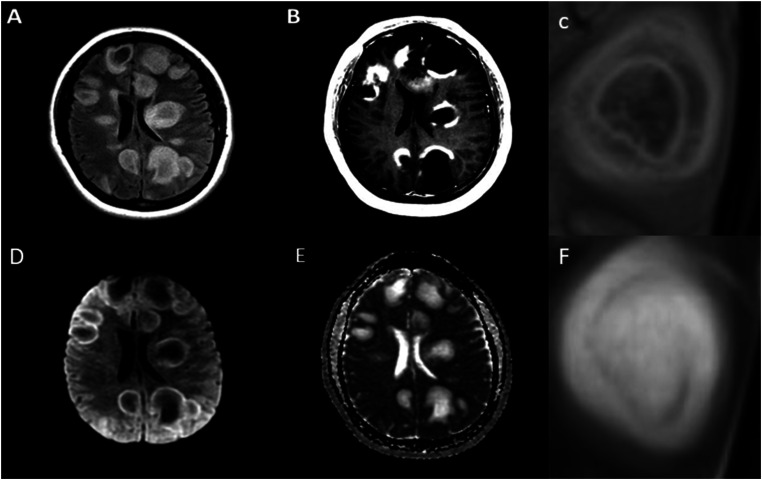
T2-weighted imaging (A) showing multiple supratentorial lesions consisting of concentric bands of alternating T2 signal intensities. T1 post-contrast imaging (B) shows peripheral ring enhancement with ‘open ring’ pattern in several lesions. Magnified T2 FLAIR imaging (C) demonstrating closer view of concentric banding. Diffusion weighted imaging (D) and apparent diffusion coefficient imaging (E) demonstrate restricted diffusion in the outer ring of several lesions. Magnified T2 weighted imaging (F) showing closer view of concentric banding.
